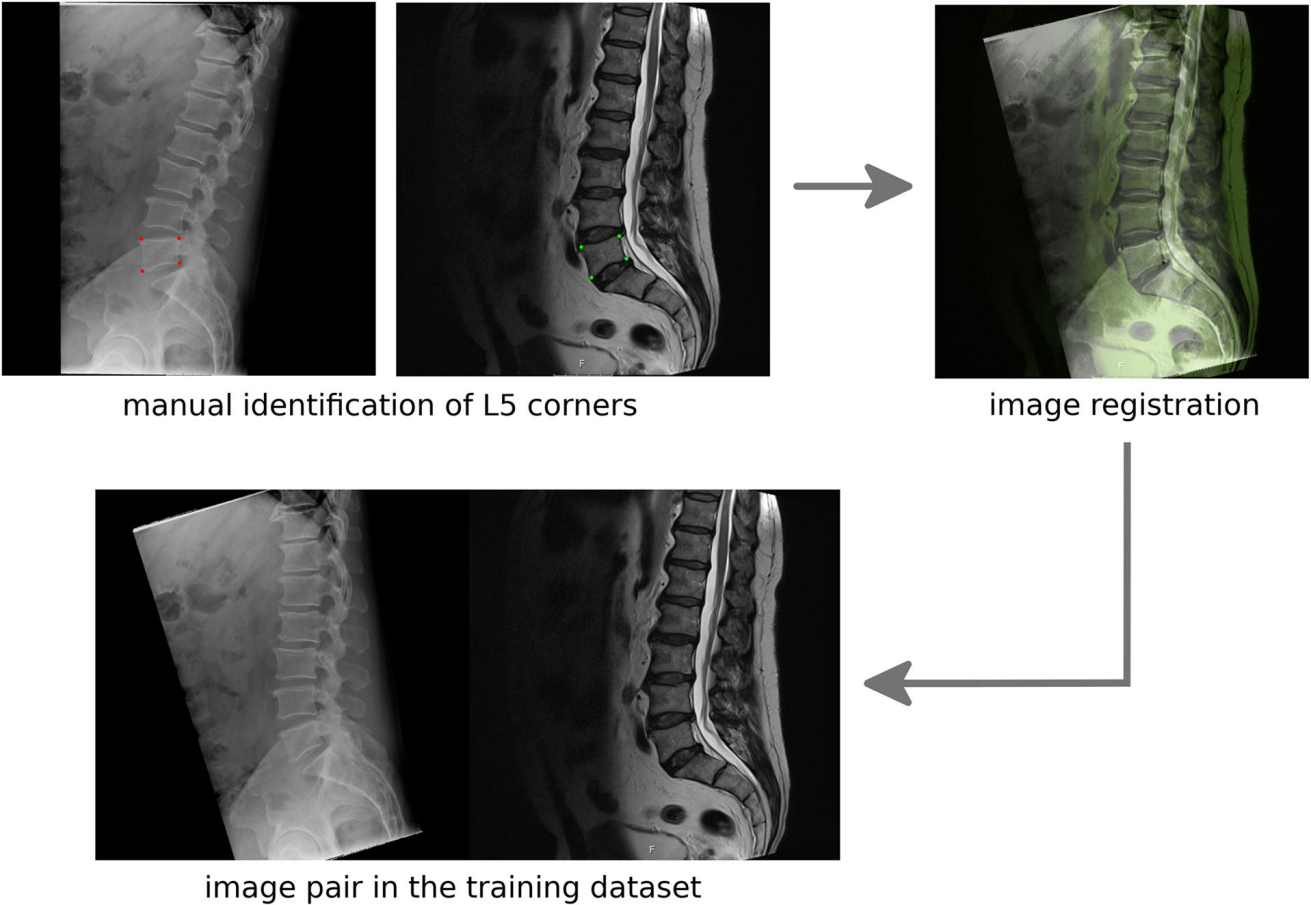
The manual registration procedure used for the alignment of the sagittal x-ray projections and the midsagittal T2W MRI scans. First, the vertebral corners of L5 are manually identified on both images. Then, a rigid registration is performed and the pair of registered images is added to the training dataset
